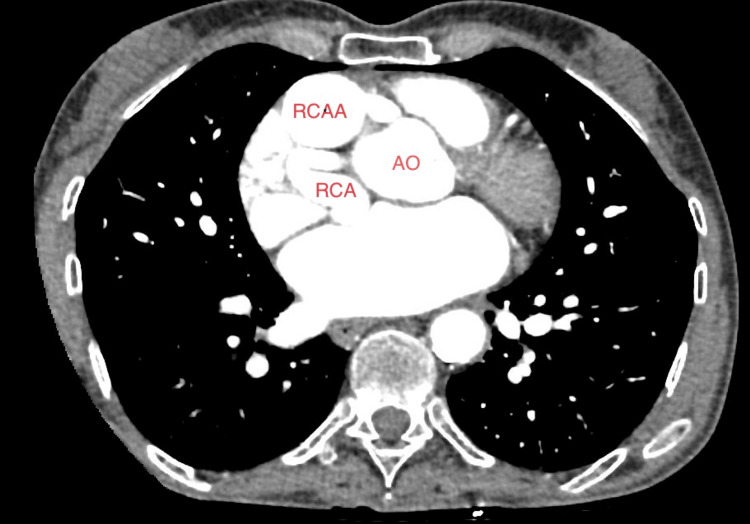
Coronary CTA demonstrating proximal RCAA. Image obtained from the patient’s chart. CTA: computed tomographic angiography; RCAA: right coronary artery aneurysm; RCA: right coronary artery
